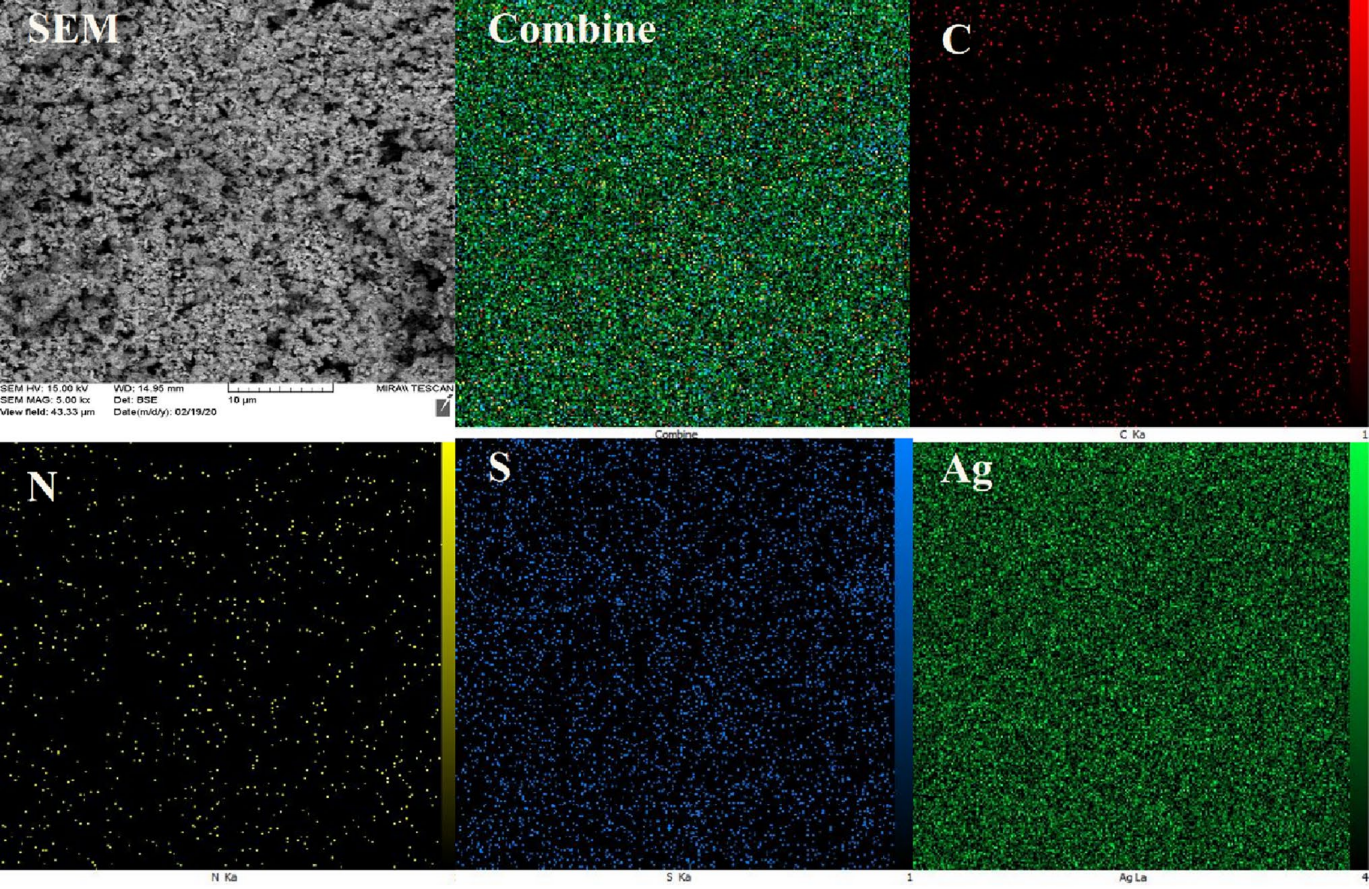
X-ray mapping Analysis of Ag–CP.
